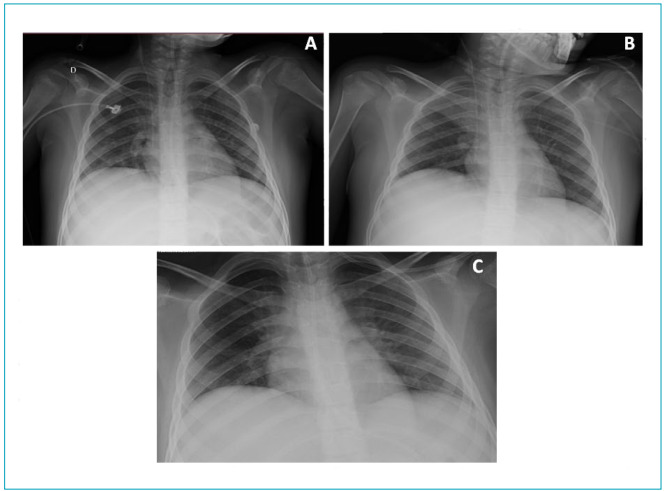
Chest radiography: (A) hypoexpanded lungs; (B) atelectasis of the right upper lobe and bilateral hypoexpansion; (C) hypoexpanded lungs.
